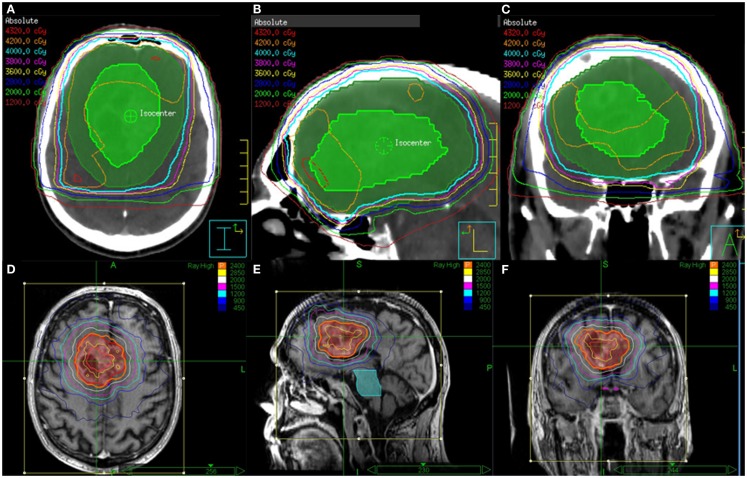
EBRT and CyberKnife SRS treatment plans for a patient who received 40 Gy in 15 fractions to FLAIR for the first course followed an SRS boost to T1 Enhancement at a total dose of 24 Gy delivered in 3 fractions. Shown are the (A) axial, (B) sagittal, and (C) coronal views of the EBRT treatment plans and the (D) axial, (E) sagittal, and (F) coronal views of the CyberKnife SRS treatment plans.
